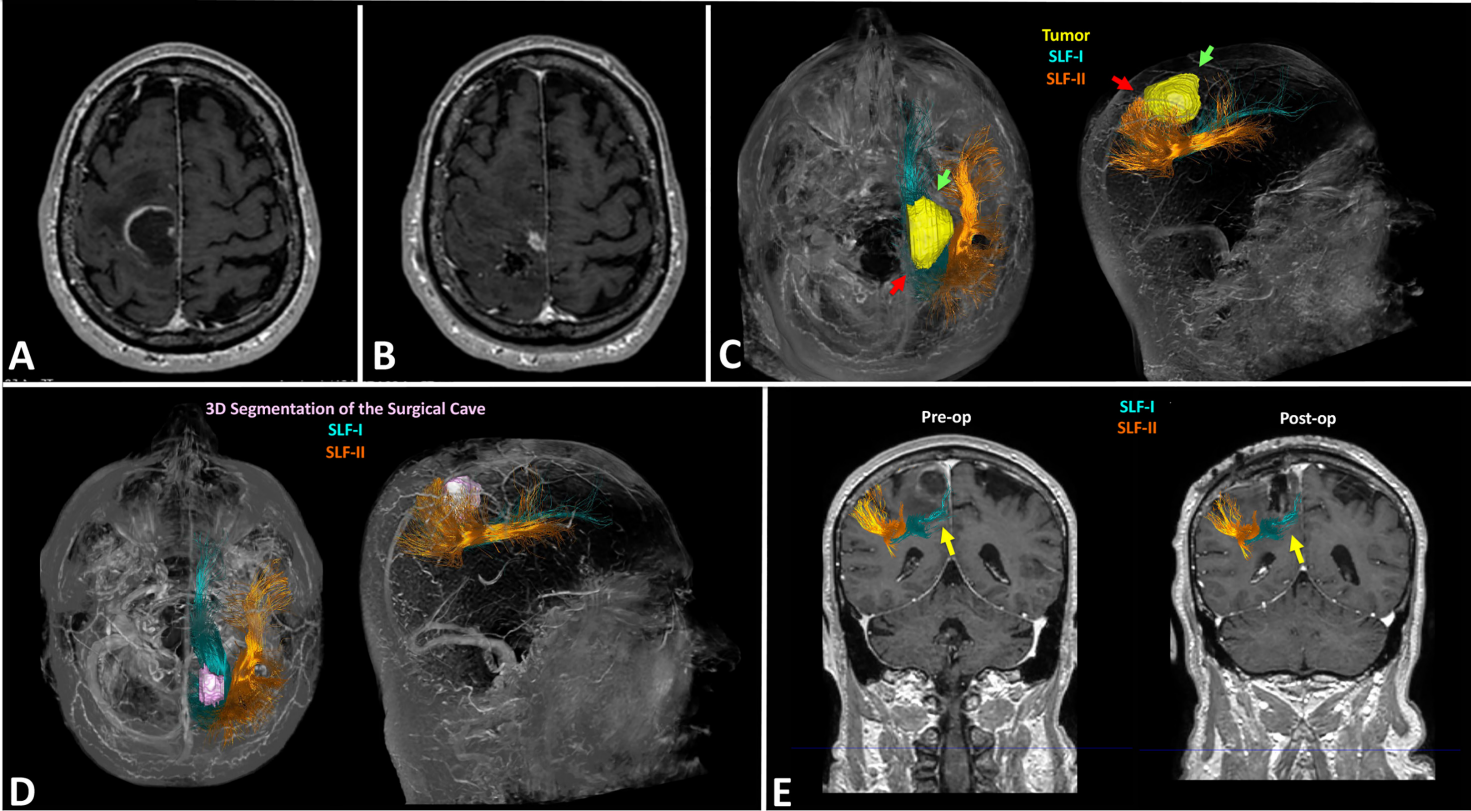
Case example of the planning in a case of right fronto-parietal GBM (case 4 in Table 1). Preoperative MRI scan documenting the lesion is located in the anterior portion of the right parietal lobe and infiltrates also the primary motor cortex (A); postoperative MRI scan documenting the subtotal resection of the lesion: neurosurgeons removed only the portion located in the right parietal lobe, while they didn’t resect the portion infiltrating the motor cortex (B); preoperative reconstruction of the VS network showing the posterior portion of the lesion is close to the blue fibers of the SLF-I indicated by the red arrows: this induced a change of surgical strategy leading neurosurgeons to start resection from the antero-lateral portion of the tumor indicated by the green arrows, just medially to the orange fibers of the SLF-II (C); postoperative DTI fiber tracking showing the 3D rendering of the surgical cave in pink, and the preserved blue fibers of the SLF-I and orange fibers of the SLF-II (D); coronal section showing the preservation of the SLF-I and II, especially the blue fibers of the SLF-I running medial to the surgical cave (E).
